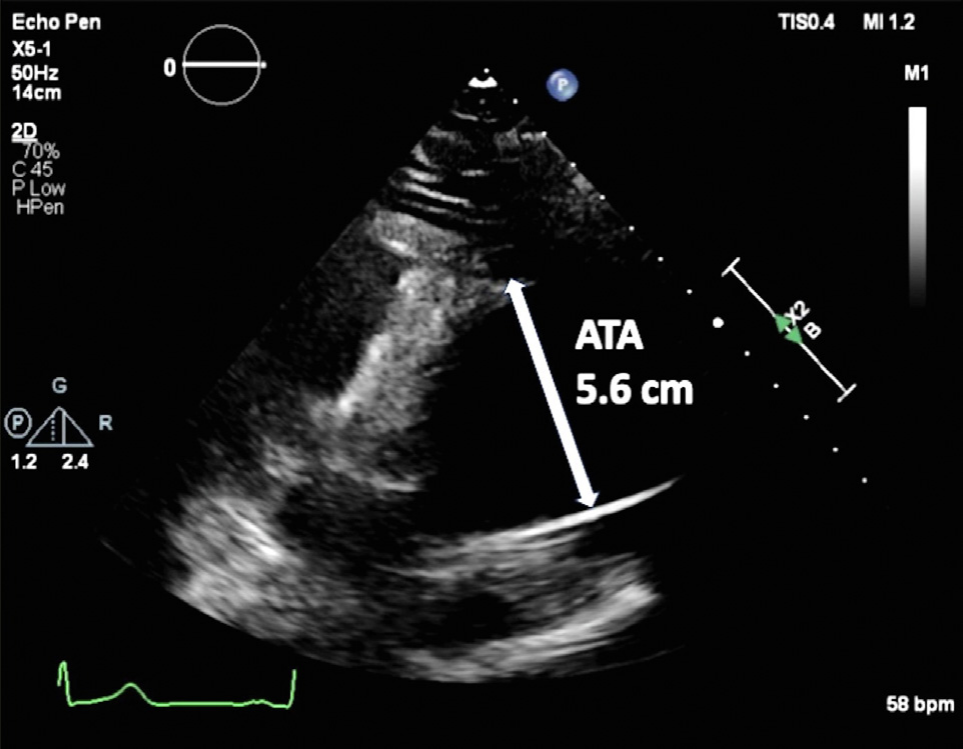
Two-dimensional TTE, modified parasternal long-axis view in diastole, demonstrates the dilated proximal ascending thoracic aorta (*arrow*) measuring 5.6 cm. *ATA*, Ascending thoracic aorta.
